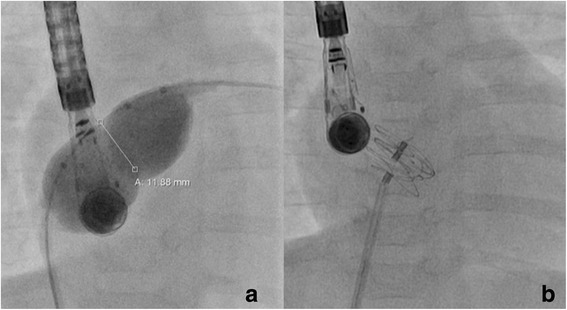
Balloon sizing of ASD and GCSO implantation. Anterior-posterior fluoroscopic view with inflated sizing ballon (“ballon sizing”) of the larger of the two ASDs while simultaneous transoesophageal echocardiography showed no residual shunting through this defect (a). Completely developed device in projection to the atrial septum, still attached to the delivery catheter (b)
